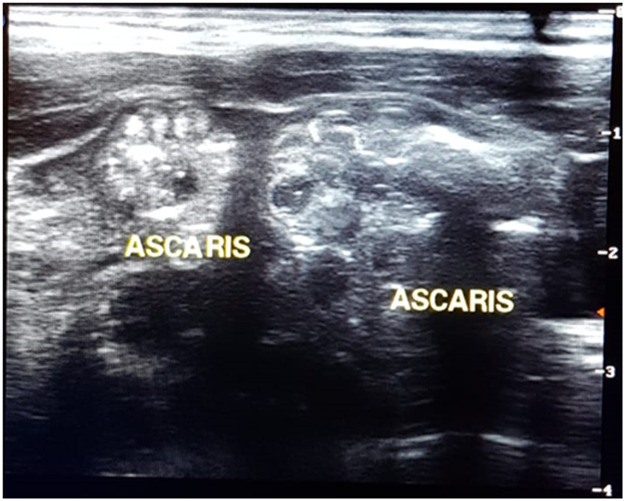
Abdominal ultrasound showing *A. lumbricoides* in the intestinal lumen.
